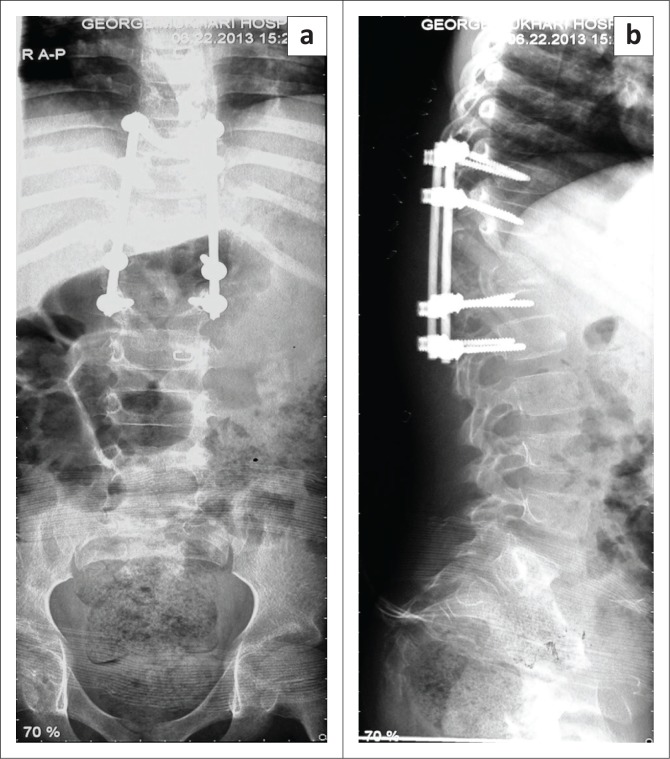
X-rays revealed intact spine post-surgery and decompression (a and b).
